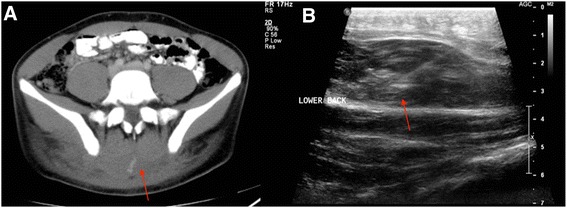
a Contrast enhanced CT of the abdomen and pelvis demonstrates a large midline paraspinal hematoma with areas of active extravasation. b Ultrasound image through the midline parasagittal hematoma demonstrating needle tip within the hematoma after thrombin injection
